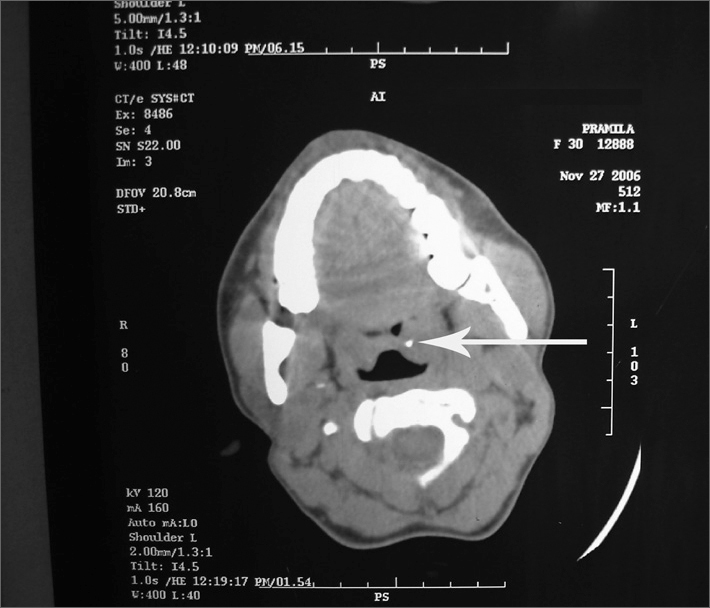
CT scan showing a small metallic density in left tonsil (arrow)
